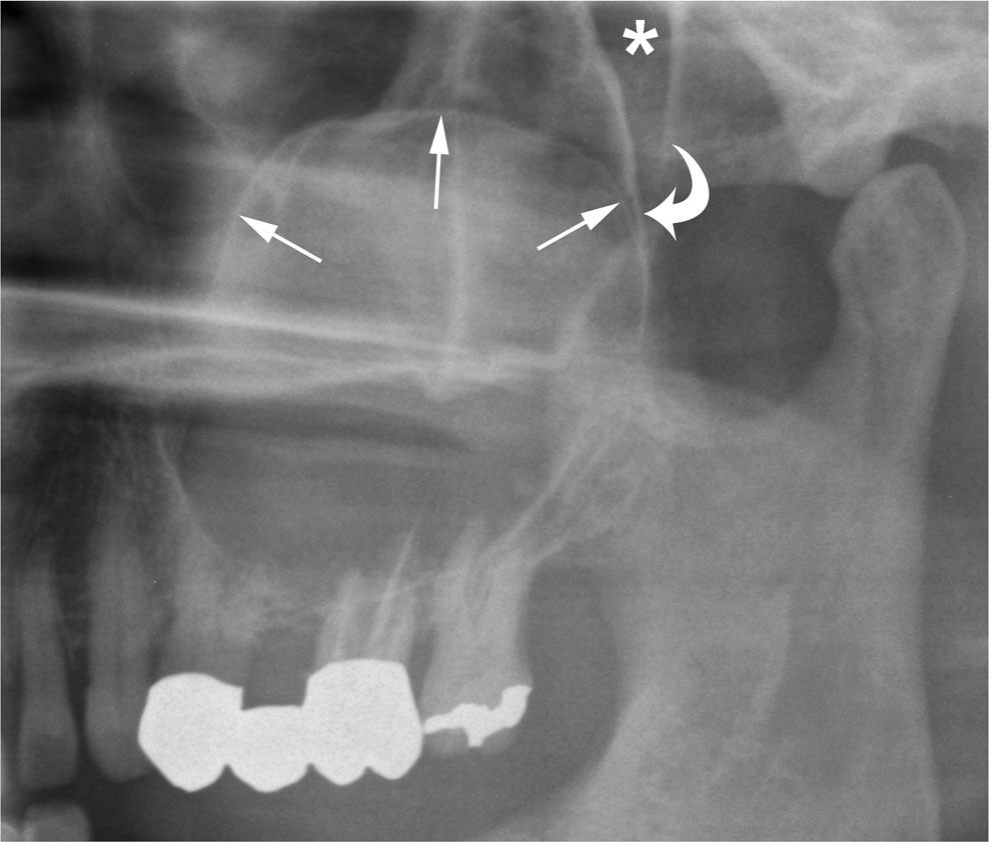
Cropped panoramic radiograph of the posterior left maxilla demonstrates a large OKC extending into the maxillary sinus (*arrows*). The posterior wall of the maxillary sinus (*curved arrow*) and the pterygopalatine fossa (*asterisk*) are also displayed
